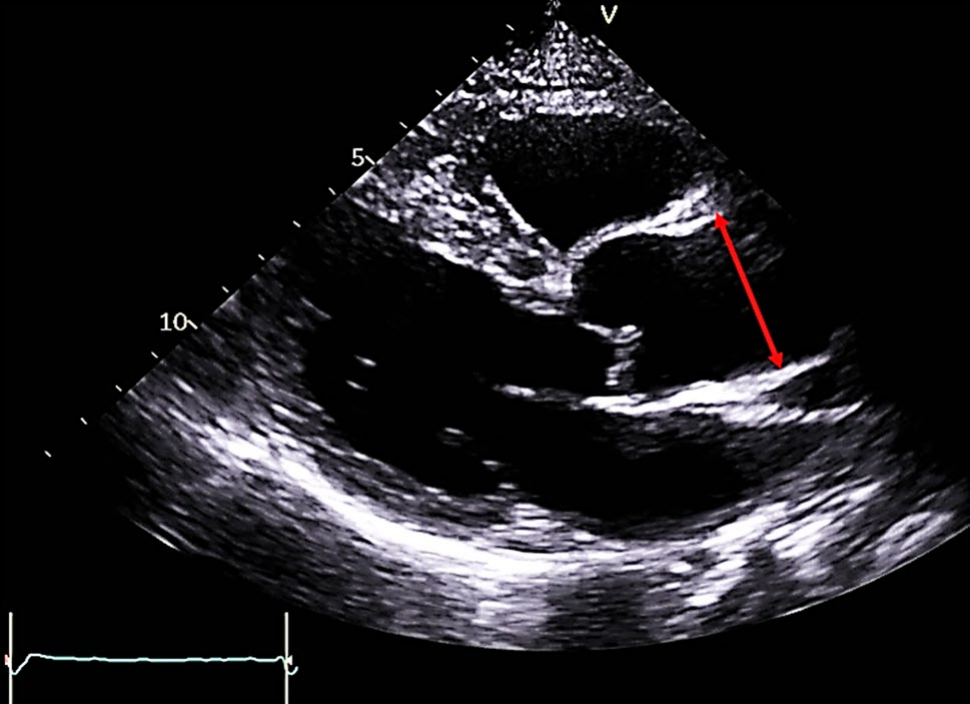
The measurement of the size of ascending aorta by transthoracic echocardiography. The measurement is taken at end-diastole in the parasternal long-axis view, with the size indicated by a red arrow.
